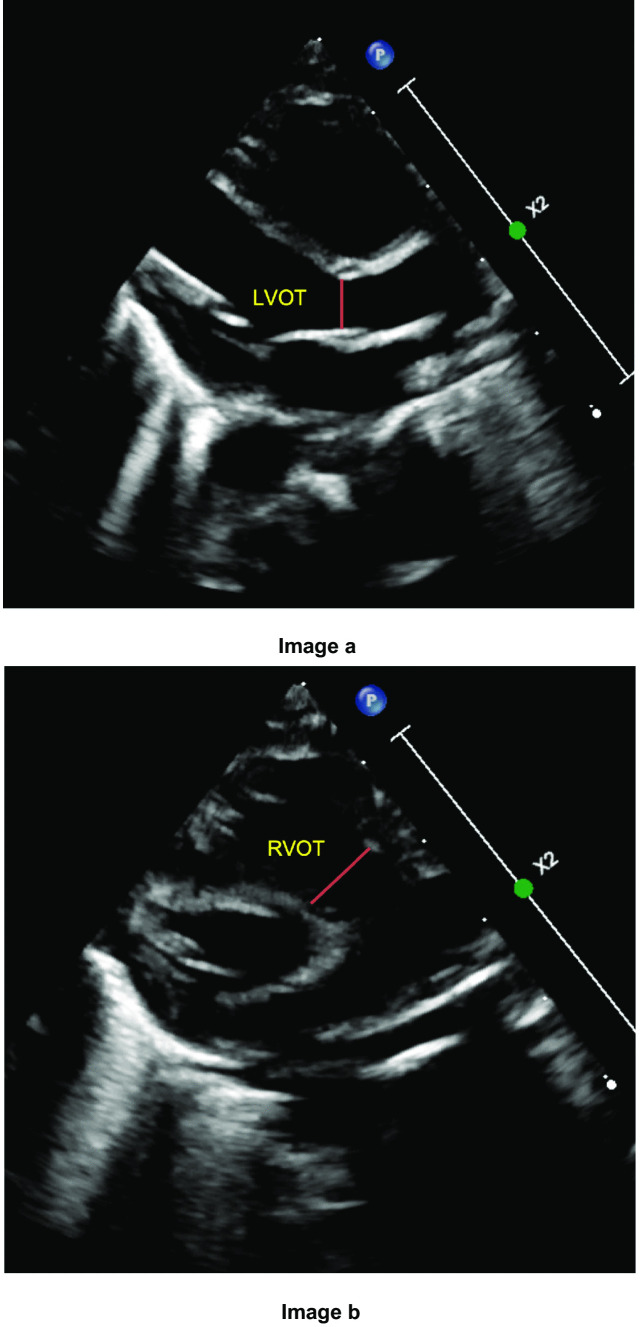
Measurement of LVOT and RVOT diameter. a shows LVOT in PLAX view with measurement of LVOT diameter at hinge point of AV valve (red line). b shows RVOT in PLAX RVOT view with measurement of RVOT diameter at hinge point of PV valve (red line). LVOT, left ventricular outflow tract. AV, aortic valve. RVOT, right ventricular outflow tract. PV, pulmonic valve. PLAX, parasternal long axis view
